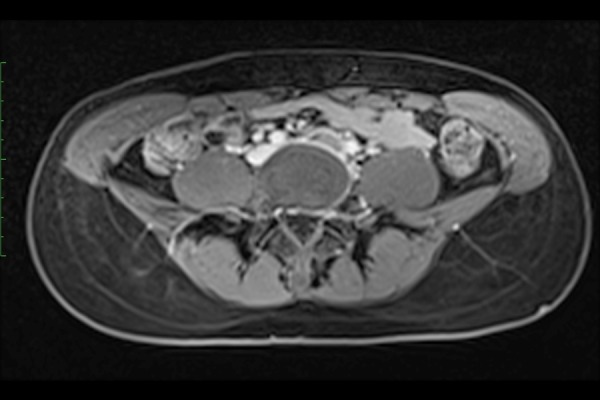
MRI of 18 year-old women showing compression and thrombotic occlusion of the left iliac vein
